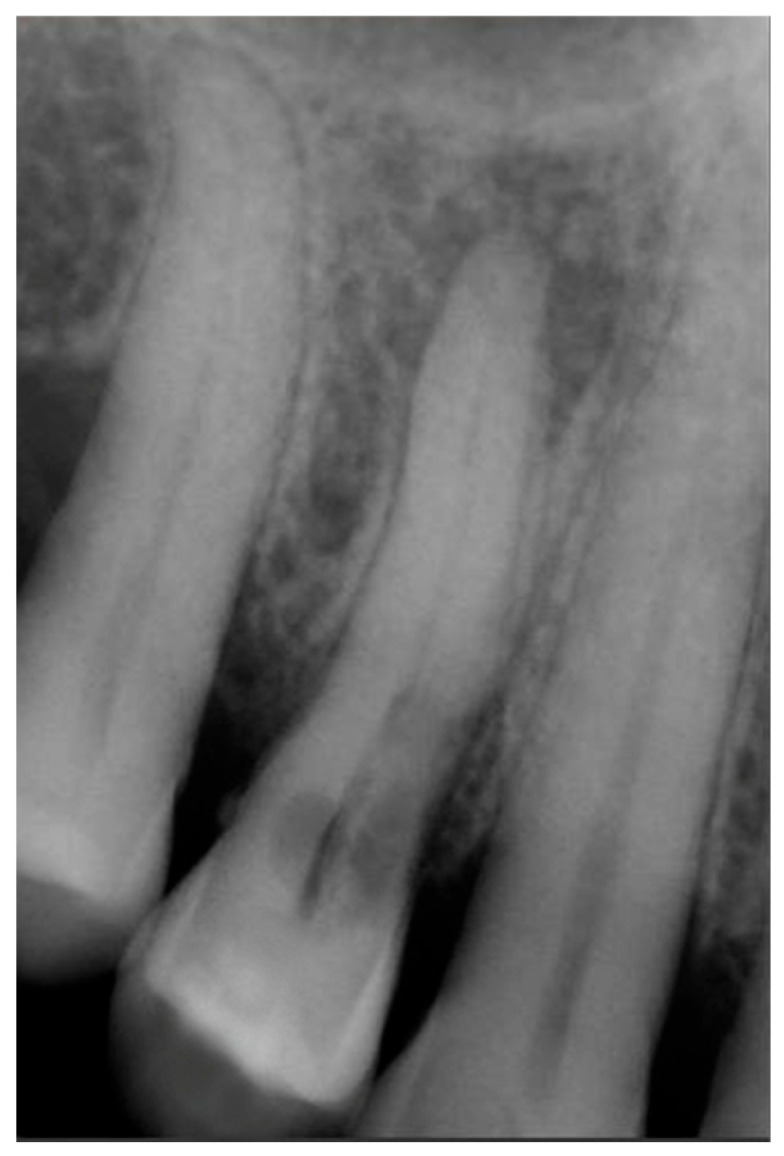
Intraoral radiography of tooth 1.4 showing root resorption, LP and EPL with mesiocervical widening of the lamina dura space.
